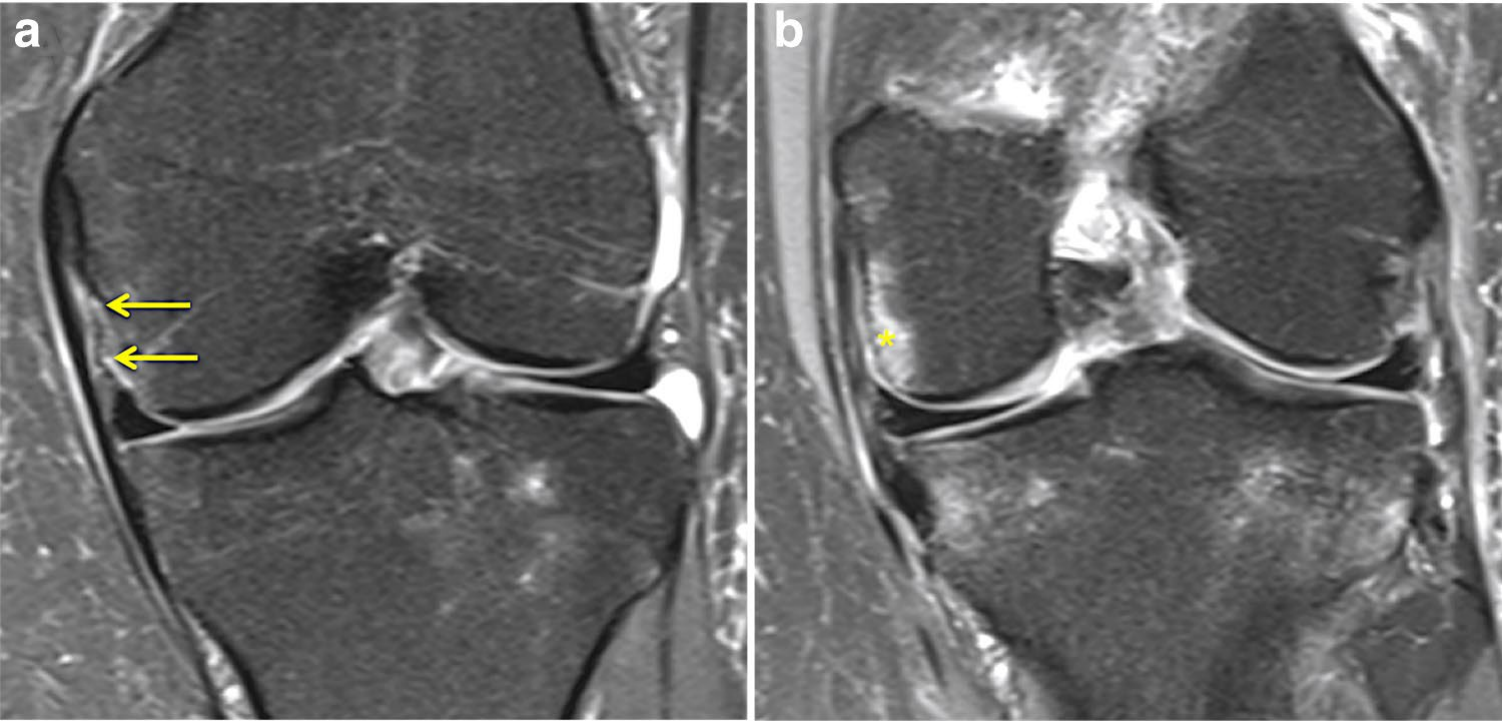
Sequential coronal fat-suppressed proton density-weighted magnetic resonance imaging shows a a meniscofemoral dMCL injury accompanying an ACL rupture with presence of joint fluid between the dMCL and the superficial MCL (yellow) and b a typical MRI bone oedema at the medial femoral condyle adjacent to the dMCL attachment site (*)
